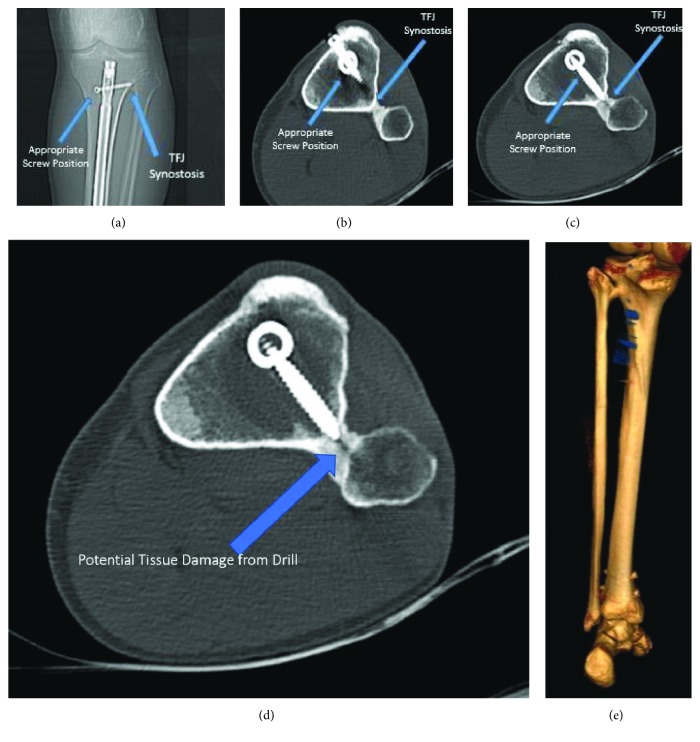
Proximal tibiofibular joint synostosis. (a) AP X-ray of the left proximal tibia show the tibial nail in an acceptable position with visible TFJ synostosis. (b) Axial CT scan showing appropriate position of the implant and TFJ synostosis. (c) Axial CT scan showing appropriate position of interlocking screw without excessive prominence. (d) CT scan demonstrating a potential soft tissue damage from drilling through the TFJ space into the fibula. (e) 3D reconstruction showing TFJ synostosis.
